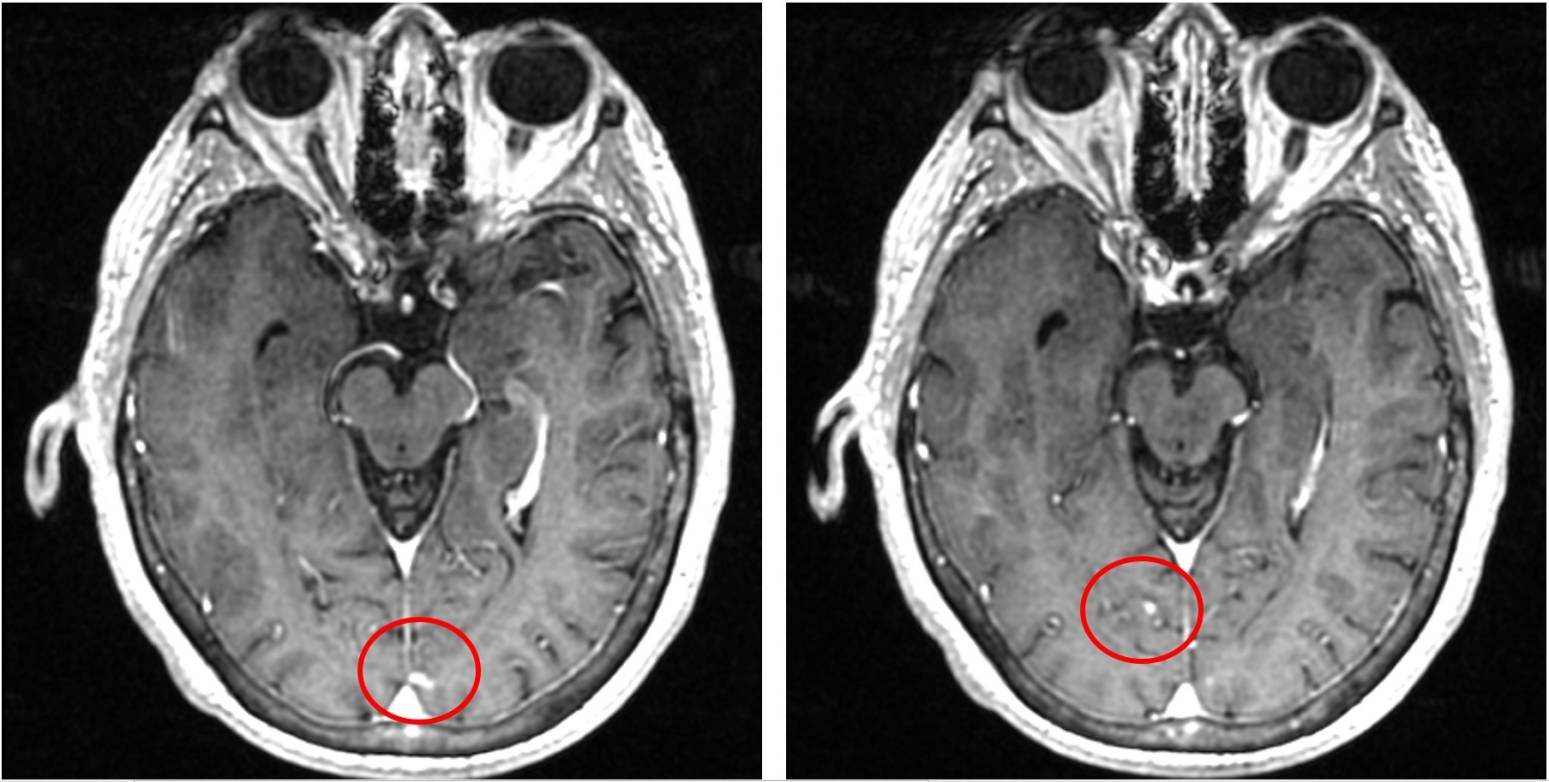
MRI of the brain showing multiple foci of superficial enhancement in the occipital region compatible with metastatic disease (red circles)
